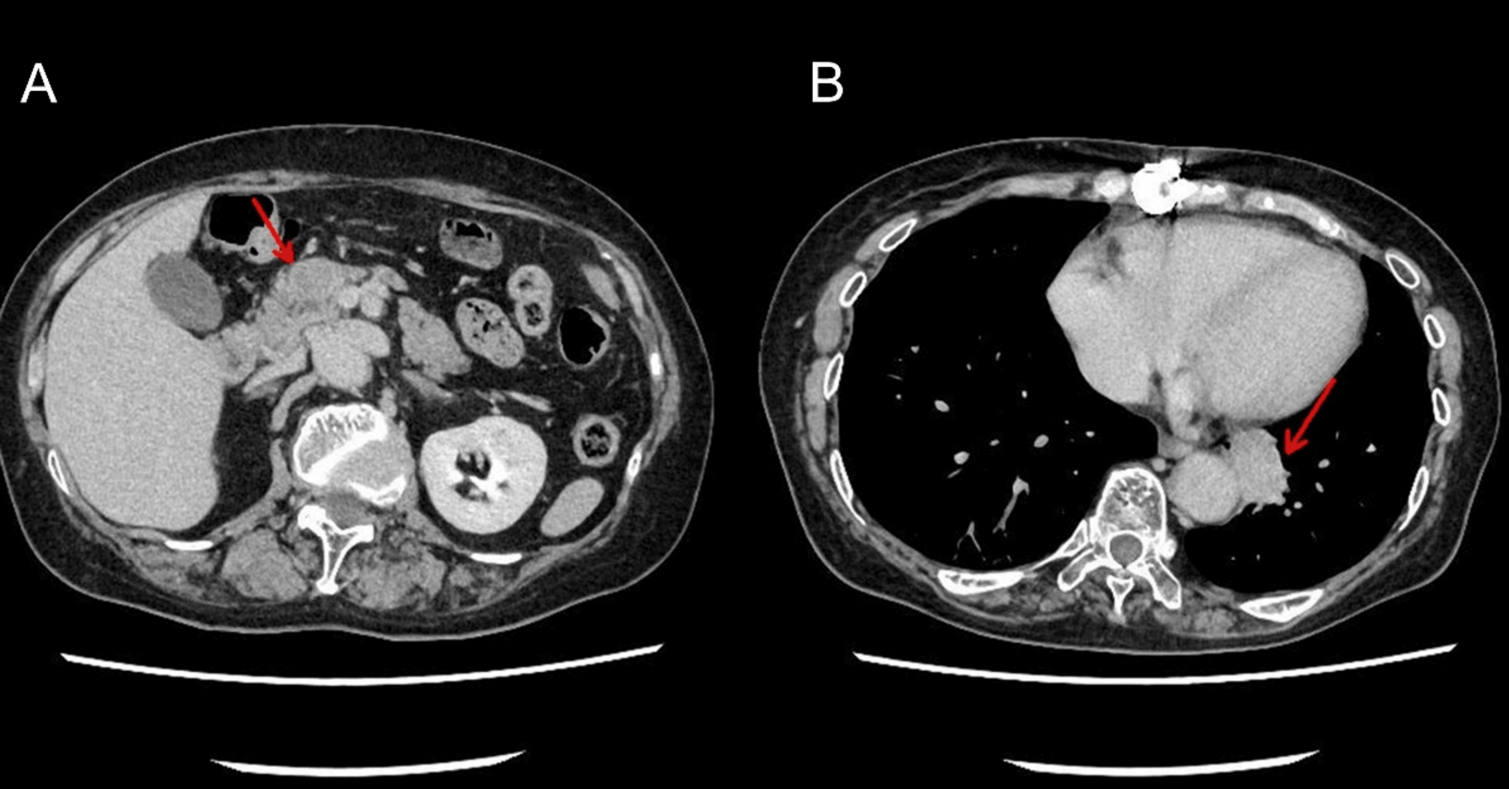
Contrast-enhanced computed tomography images. (A) Axial image showing a 20-mm mass protruding from the ventral aspect of the pancreatic head (arrow). (B) Axial image demonstrating a lobulated mass in the left lower lobe of the lung adjacent to the aorta (arrow).
